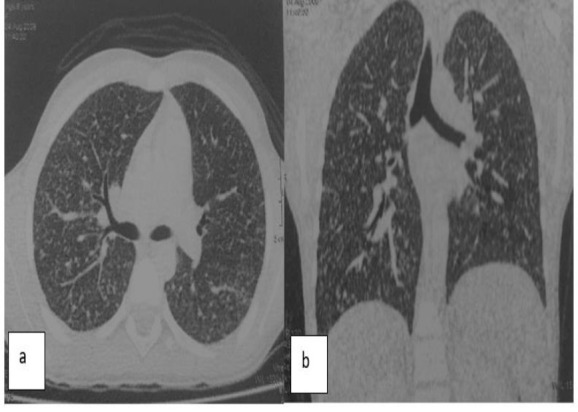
Chest CT scan in axial and coronal sections showing miliary carcinomatosis.
